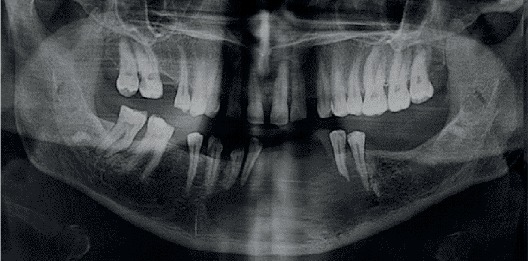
The panoramic radiograph shows an ill-defined unilocular radiolucency extending from the right lateral incisor to the left second premolar, as well as root resorption of teeth 20, 21, and 22.
